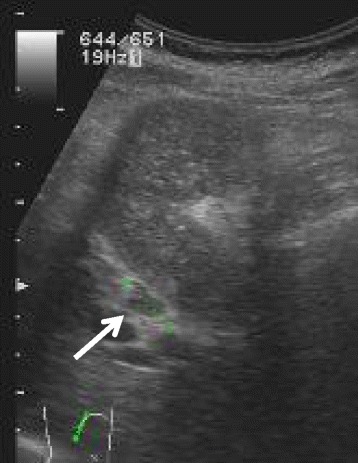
Ultrasound sonography showed enlarged gallbladder filled with concentrated bile juice and enlarged lymph node along the GB wall. The wall thickness was not detected
